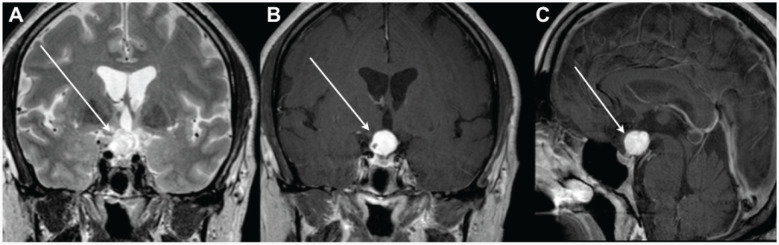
Brain magnetic resonance imaging showing a large suprasellar contrast-enhancing hemangioblastoma. (A) T2-weighted image, coronal view; (B) T1-weighted image, coronal view; (C) T1-weighted image, sagittal view.
